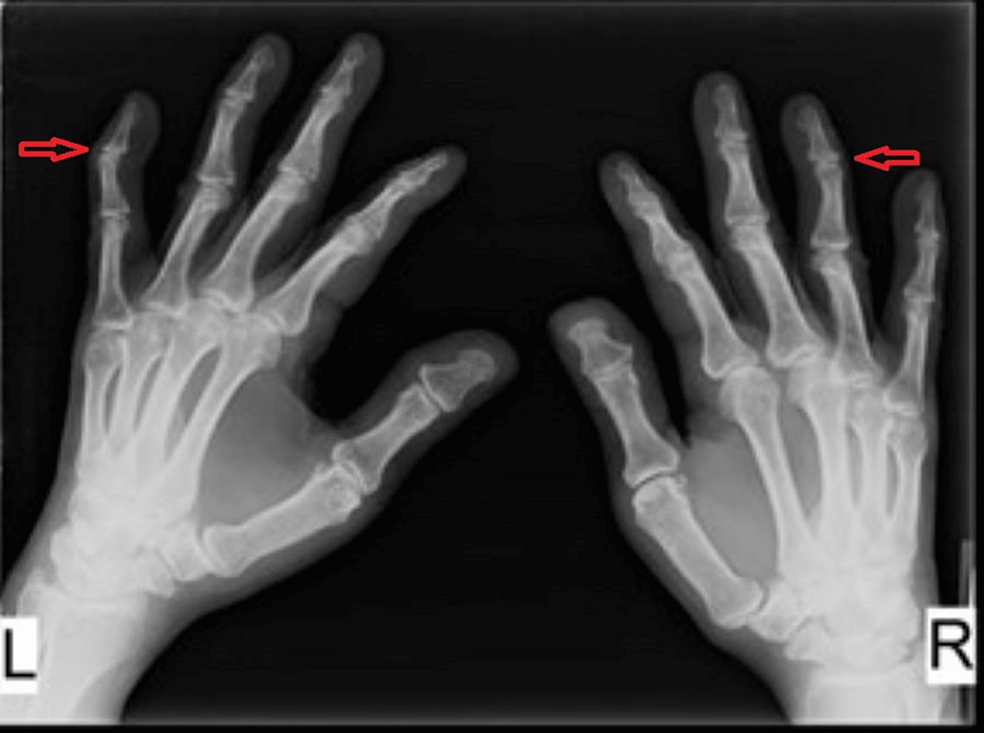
Radiograph of the hands showing flexion of the terminal phalanx of the 5th finger of the left hand with mild thickening of extensor soft tissue at the level of distal phalanx of the left 5th finger.
